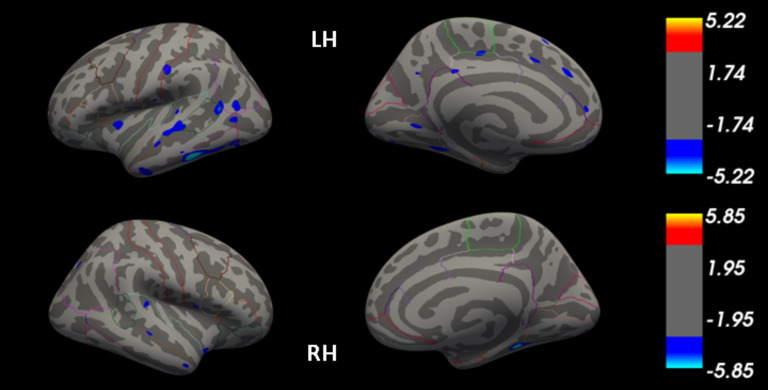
Association of neurofilament light (NF-L) levels with cortical thickness (CT) in patients with anorexia nervosa (AN). FDR-corrected statistical maps (*q* < 0.05) displaying regions in which log-transformed NF-L levels are associated with CT plotted on the inflated surface of a standard average subject. The color scale shows *p*-values expressed as –log10(p). Cool colors indicate a negative association between CT and NF-L. Abbreviations: LH, left hemisphere; RH, right hemisphere. Colored outlines correspond to anatomical labels of the Desikan-Killiany atlas (Desikan et al., 2006).
